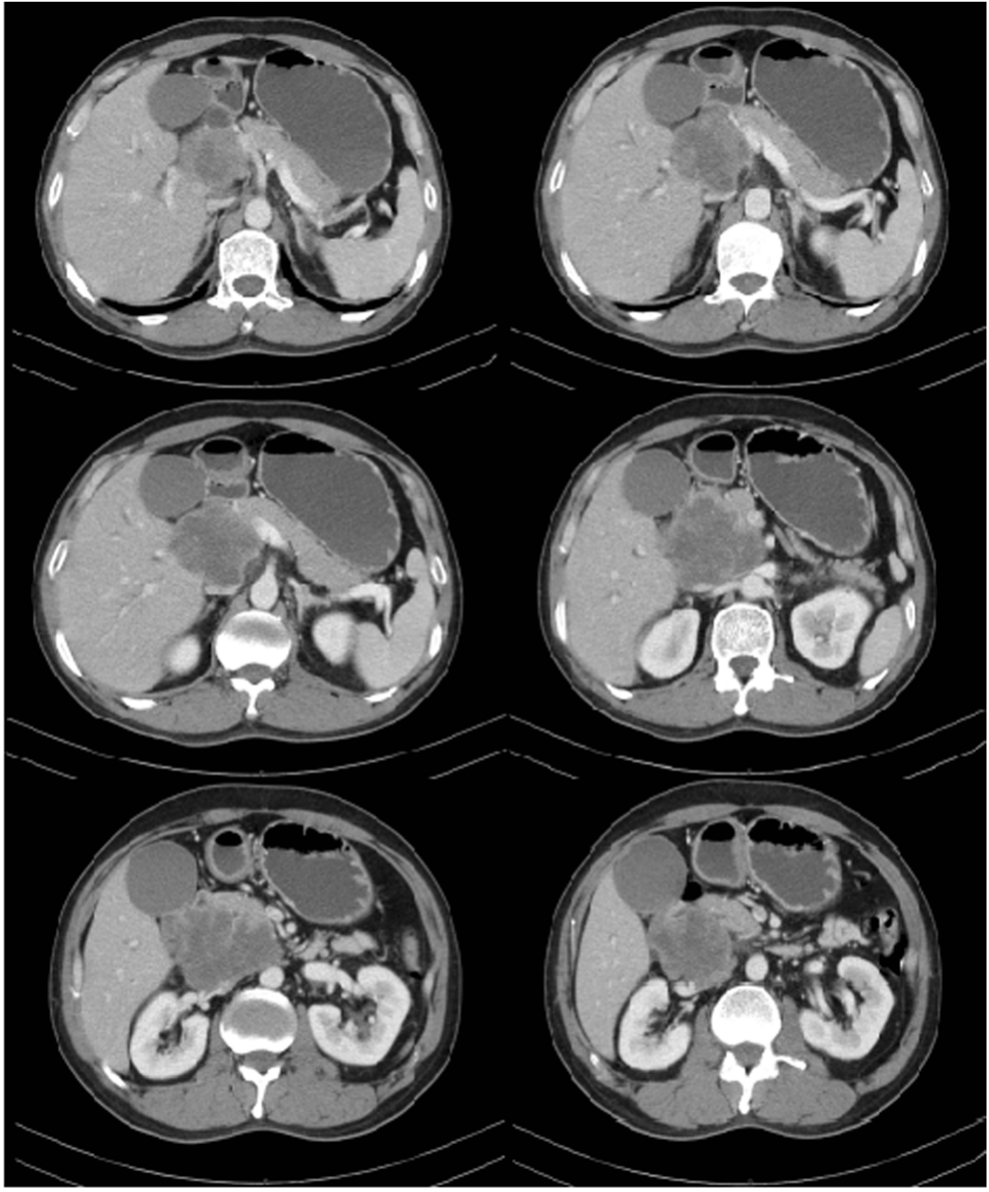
CT: The enhancement of tumor is inhomogeneous, has fuzzy boundaries, and involved the common bile duct, duodenum. The tumor was adjacent to the portal vein, the superior mesenteric vein, the inferior vena cava, and the right renal vein
